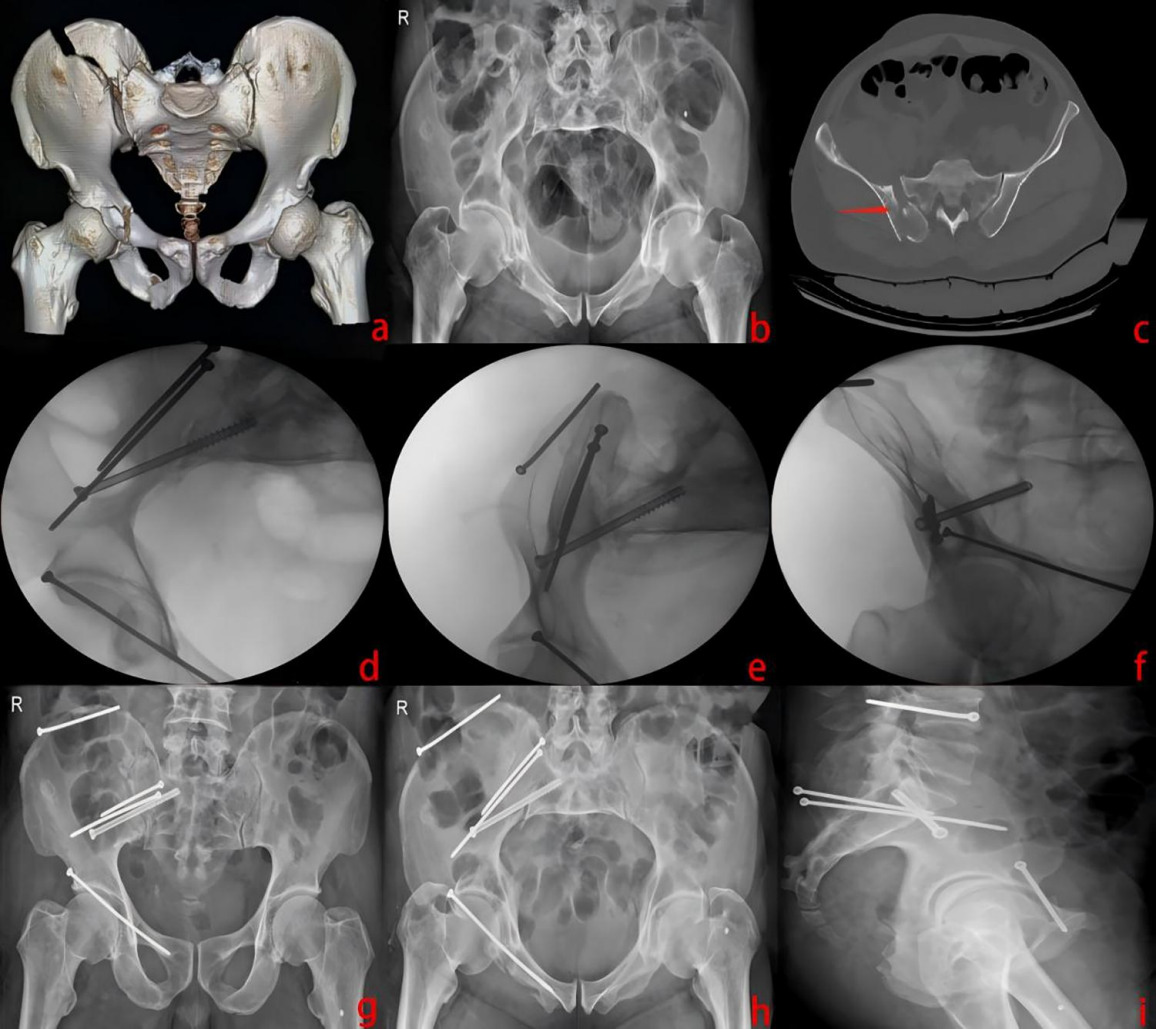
Pre-operative CT and x-ray images of a 58-year-old male patient with a Day type II CFDP (a–c). Surgery was performed with the patient in the prone position. Small incisions were made over the posterior superior iliac spine (PSIS) and the right iliac crest to reduce the iliac fracture. Two LC-II screws were inserted through the PSIS for fixation, followed by placement of a modified sacroiliac screw. Finally, the right superior pubic ramus was fixed with an anterior column screw (d–f). Postoperative pelvic radiographs demonstrated satisfactory fracture reduction and appropriate implant positions (g–i).
